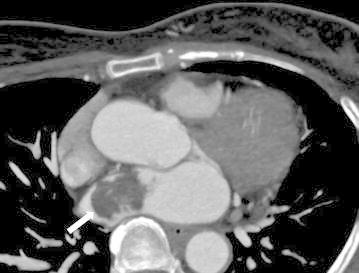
Coronary computed tomography angiogram. Large mass is located in the left atrium and appears heterogeneously low attenuating. The point of attachment appears to be the interatrial septum
